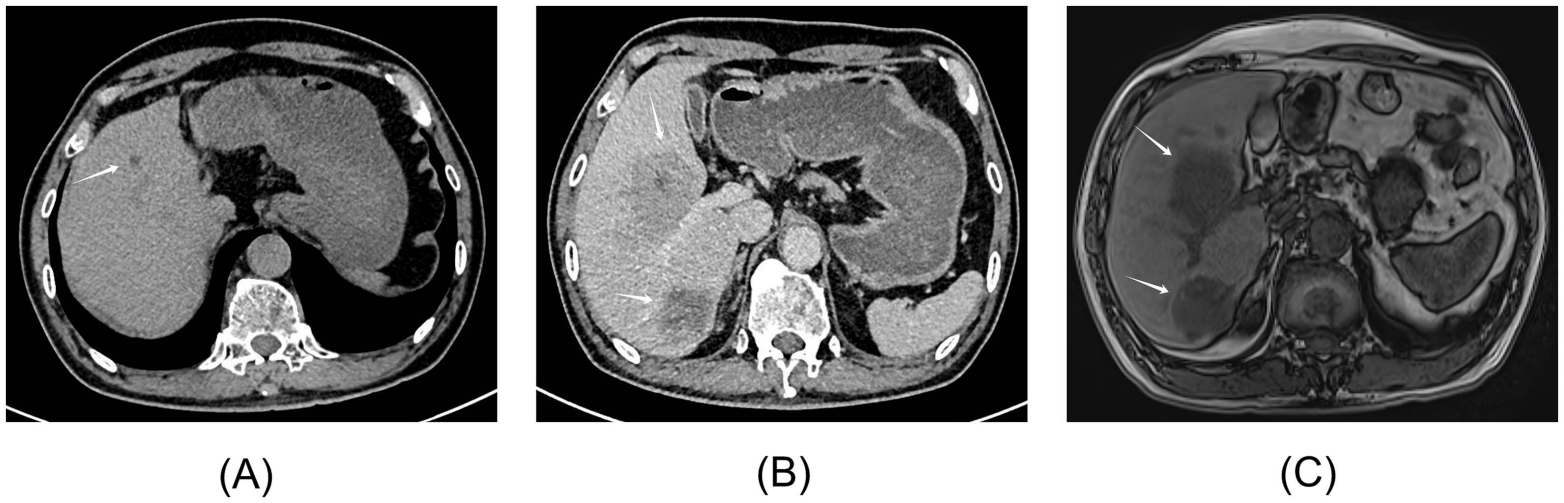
Imaging findings of Case 1. (A) Unenhanced and contrast-enhanced CT of the liver in September 2023. A low-density lesion of approximately 2.3 × 2.1 × 1.4 cm in the right lobe of the liver was considered an “atypical hepatic hemangioma.” (B) Contrast-enhanced CT of the upper abdomen in September 2024. Low-density, circular shadows with relatively clear boundaries were observed in liver segments S5 and S6. The larger lesion was approximately 8.8 × 8.2 cm. Enhanced scanning showed marginal enhancement of the lesion in the arterial phase and continuous enhancement of the lesion in the venous and delayed phases. (C) Upper abdominal MRI in September 2024, including unenhanced, contrast-enhanced, DWI sequences, and magnetic resonance cholangiopancreatography. Space-occupying lesions in liver segments S5 and S6 measured 10.0 × 8.0 cm and 4.4 × 3.8 cm, respectively. The lymph nodes in the hepatic portal area were enlarged.
